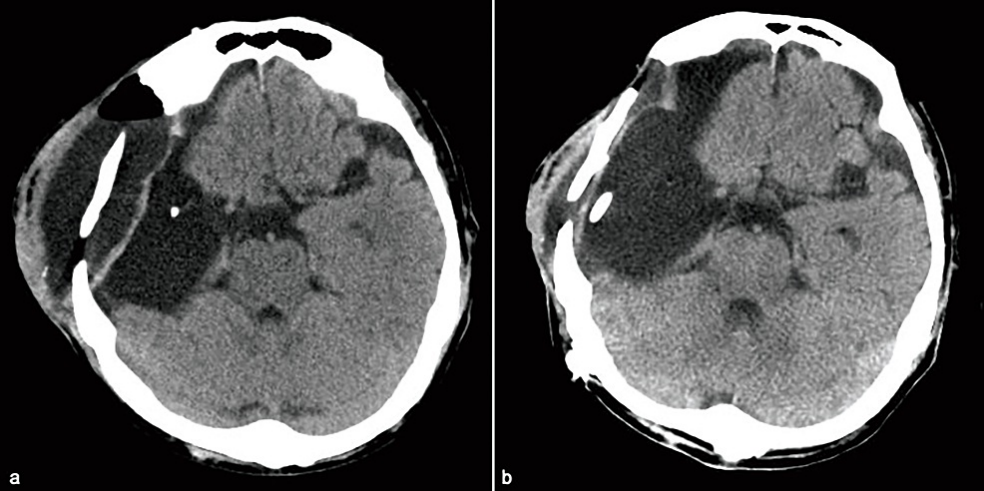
Postoperative CT after removal of Giliadel wafer implants (a) CT showing the CSF leakage remaining for three weeks even after removal of the Gliadel wafer implants. (b) A subdural-peritoneal shunt was performed three months after the primary tumor resection, and the CSF leak was successfully resolved.
